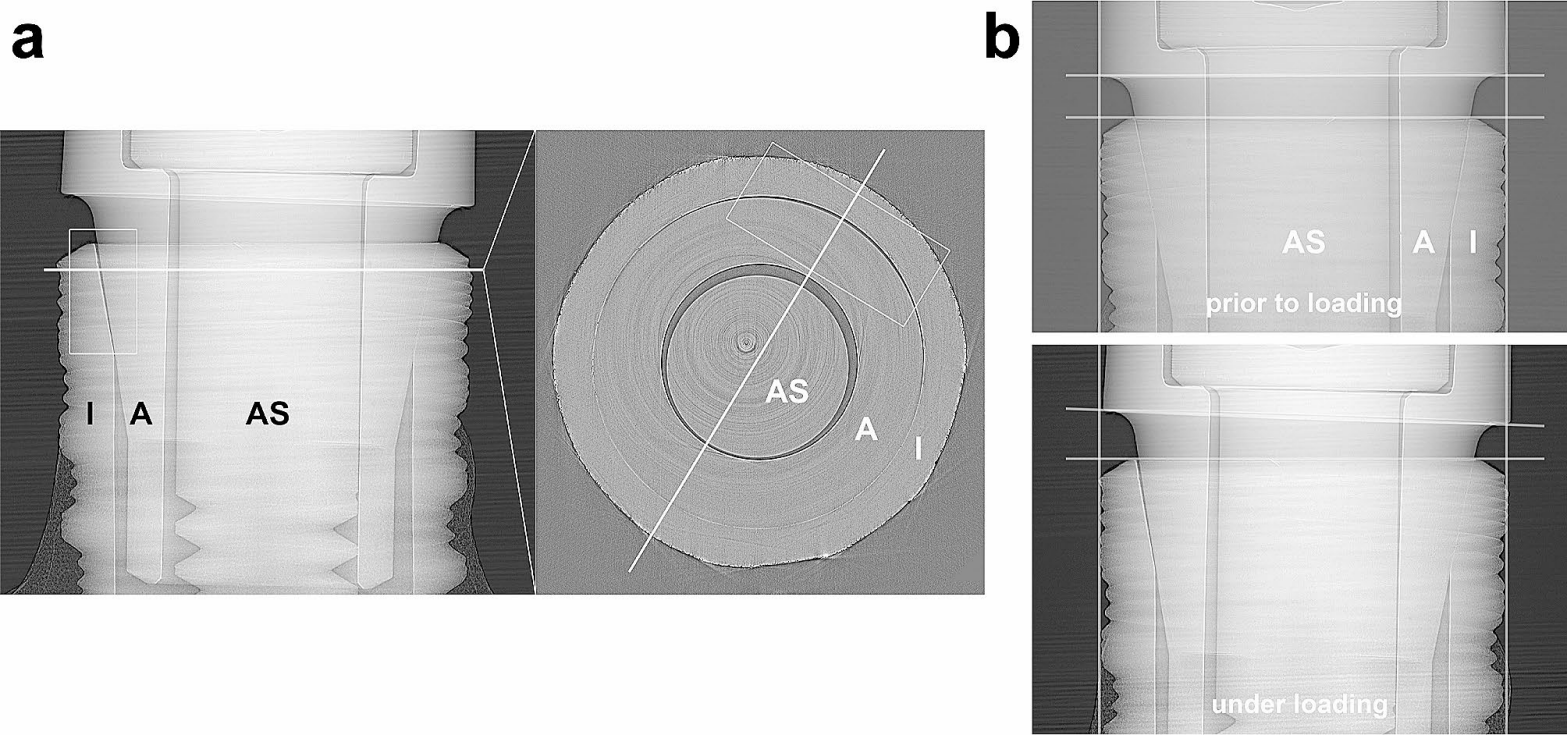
Synchrotron-based microtomography of microgap formation in MA implant. (I: Implant; A: Abutment; AS: Abutment screw); a: Even after force release a large microgap remains on the application side of the IAC. The azimuthal extension of the gap can be observed from horizontal micro-tomography slices (level of the slice is indicated by horizontal white line in the left hand tile, the diagonal white line in the right hand tile indicates the direction of previous load application) The side of application displays a sickle-shaped microgap (box in the right hand tile); b: Additional observation from radiography: off-axis loading leads to tilting abutment movement inside IAC and slight lateral implant wall deformation visible at right white positioning line
